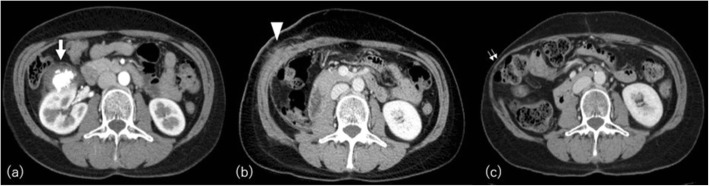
A 59-year-old woman with post-surgery lipoatrophy. a CT image before operation shows calcified soft tissue density mass anterior to the right kidney (arrow). Right nephrectomy was done, and the lesion was pathologically diagnosed as dedifferentiated liposarcoma. b CT image one month after surgery shows fluffy opacity in the subcutaneous fat around the operated area (arrowhead) with abdominal wall muscle swelling. c CT image after 8 years shows local lipoatrophy (double arrow) with muscle atrophy
